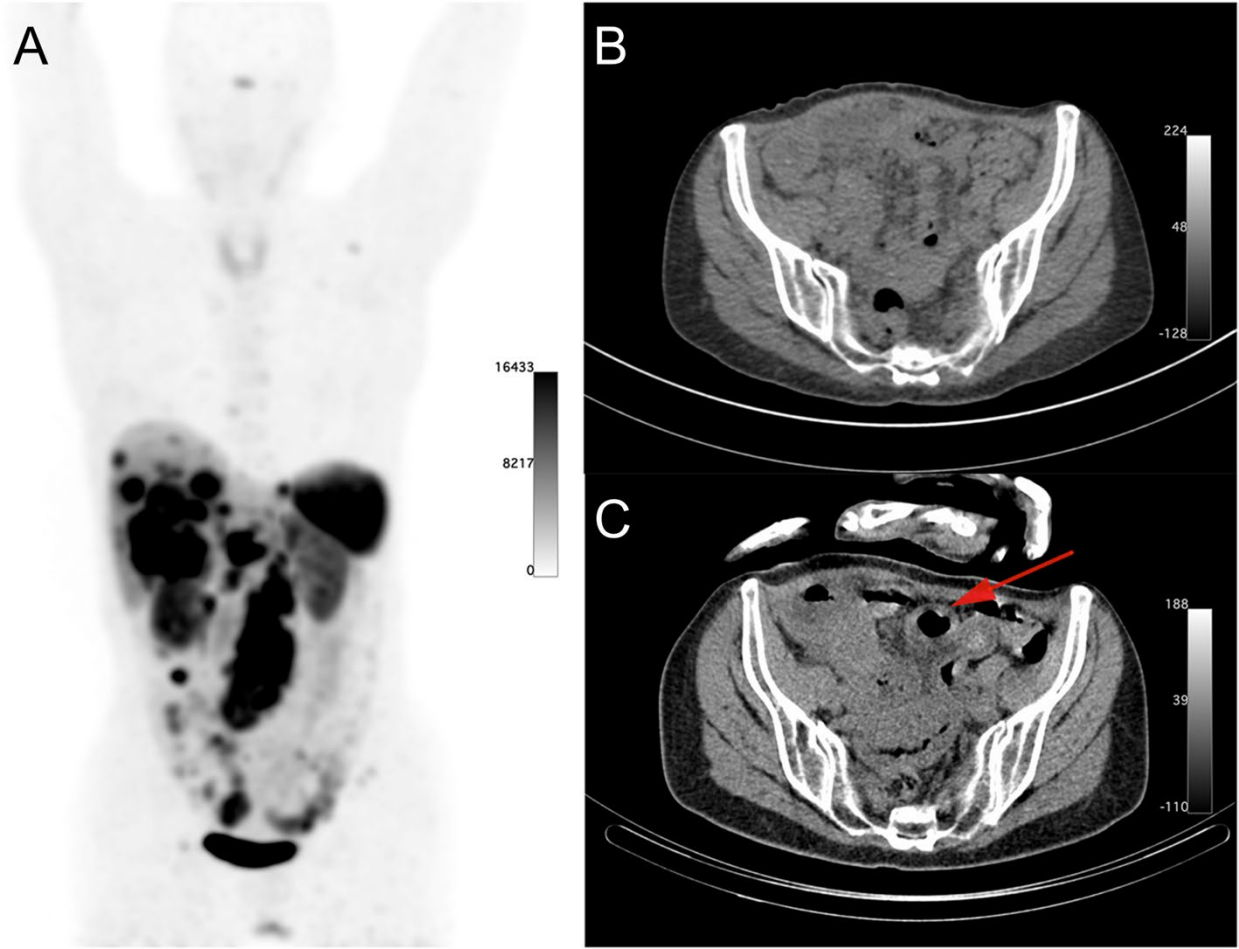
Maximum intensity projection of pre-therapy [^68^ Ga]Ga-HA-DOTATATE PET/CT (A). The pre-therapy CT showed no abnormalities (B), but after the third PRRT cycle swelling of the intestinal wall can be seen on the CT scan (C)
